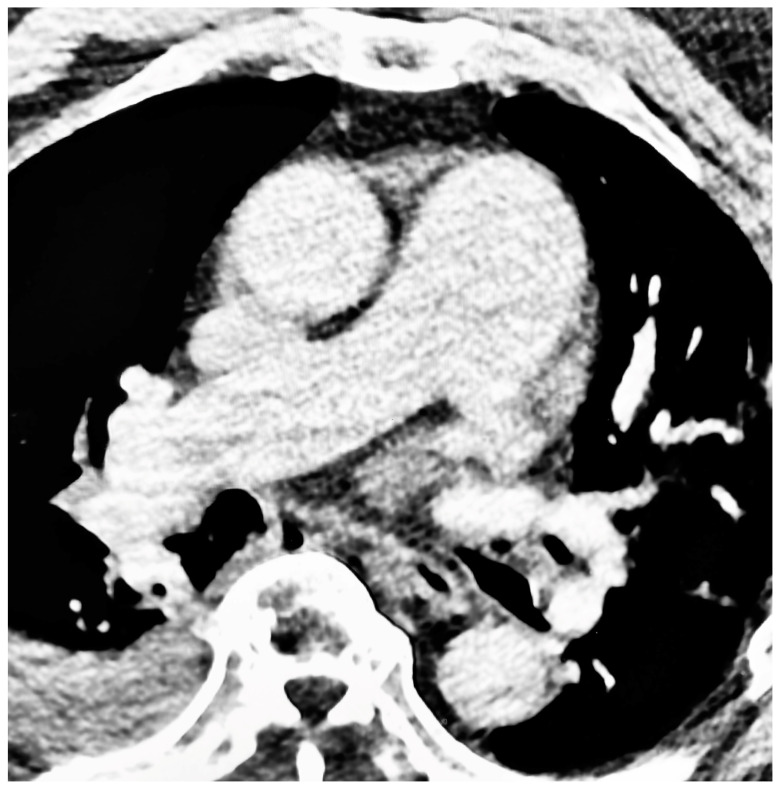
Pulmonary hypertension in a patient with sleep apnea. Axial, contrast-enhanced CT scan of the thorax with a section taken at the level of the main pulmonary artery bifurcation. The main pulmonary artery has a width of 49 mm. Incidental note is made of a moderate right pleural effusion.
